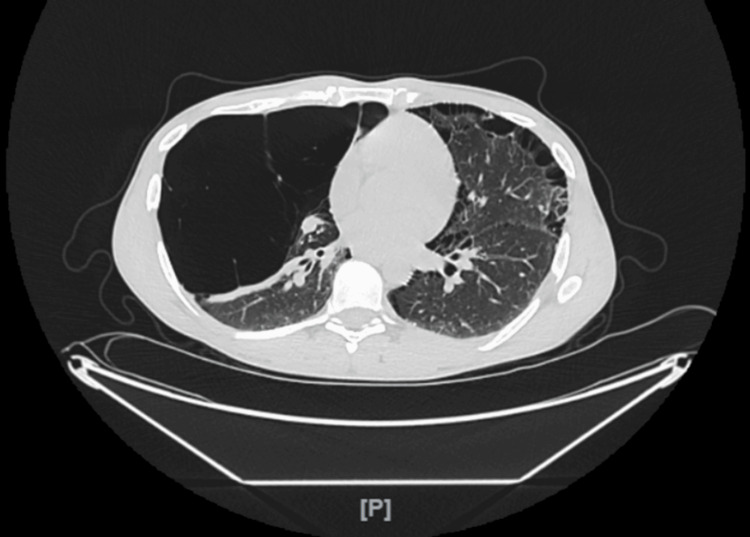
Chest CT scan transverse view showing severe bilateral apical bullous emphysema without pneumothorax.
